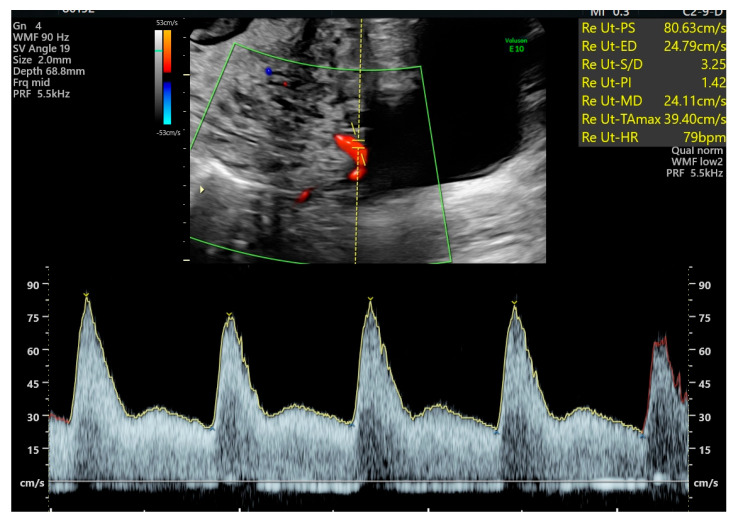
Uterine Artery Doppler: transabdominal measurement with a hint of a notch.
